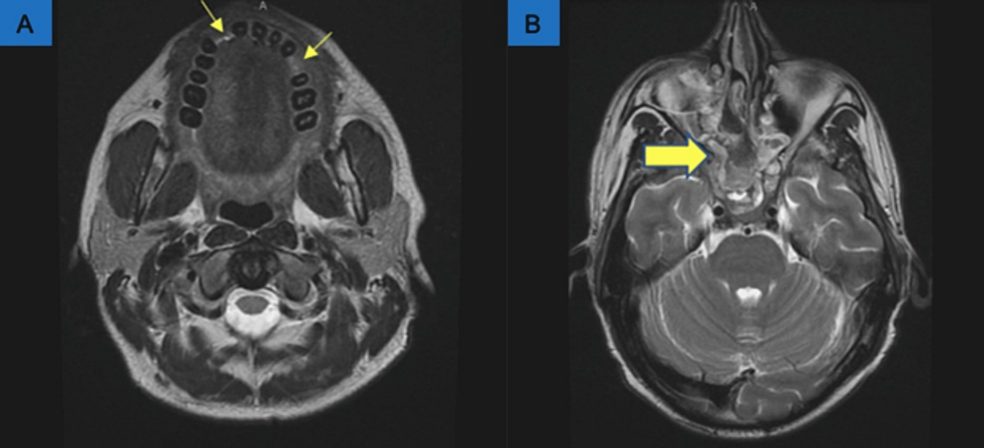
Repeat MRI orbit face and neck (without contrast) on day 8 of admission showing now absent dentition (thin yellow arrows) as well as postoperative changes (thick yellow arrow).
